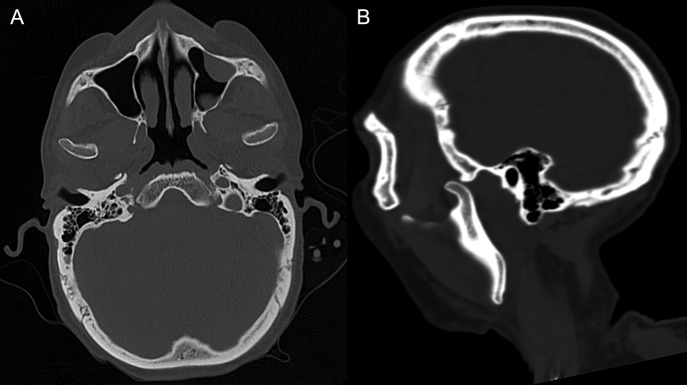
CT of the craniofacial skeleton without contrast showing the bilateral dislocation of mandibular condyles. (A) Shows as axial section and (B) sagittal section.
